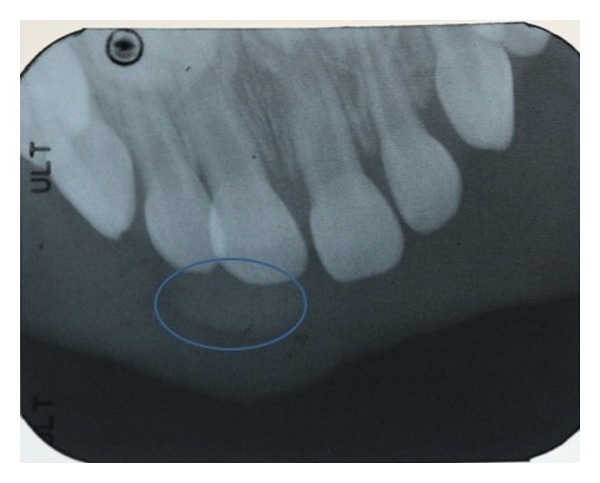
Radiographic image with palatal lesion circled.
